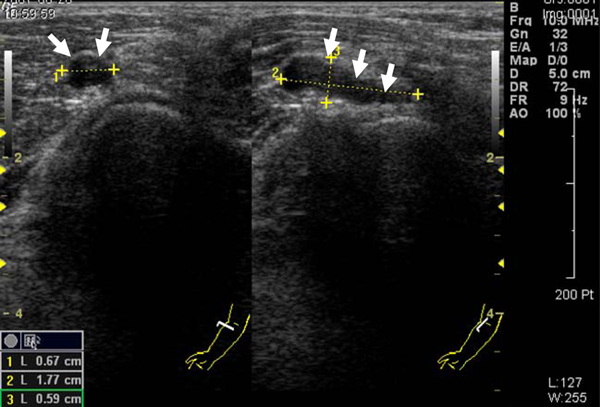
Sonogram showing a cystic lesion reveals a 0.6 × 0.7 × 1.8 cm hypoechoic mass that is just anterolateral to the radial head. The cystic lesion is likely causing a mass effect against the sensory branch of the radial nerve.
